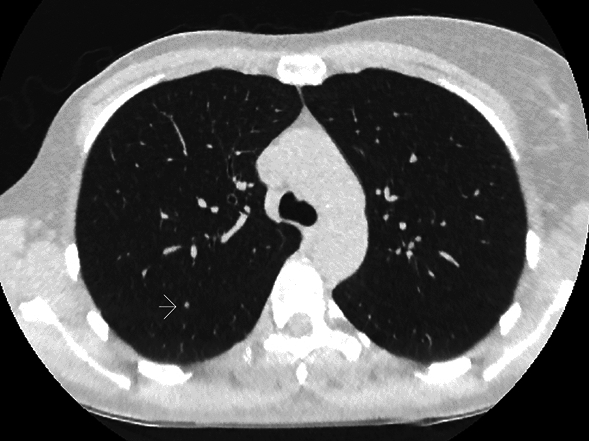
Ultra-low-dose CT (ULD-CT) image of a patient for whom radiologist considered the ULD-CT finding diagnostic and verification with normal dose contrast enhanced CT unnecessary. Slice shows one of the four metastatic nodules of all which were invisible in chest X-ray.
